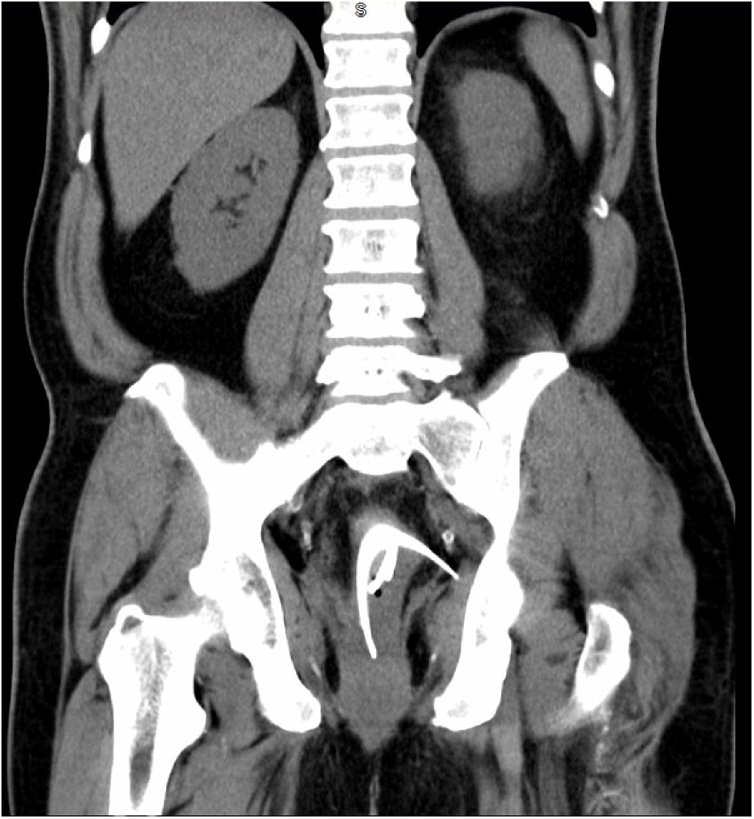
Coronal CT revealing multiple fragments of glass. One portion of the glass appeared to be traversing the inferior left lateral rectal wall, which was suspicious for perforation, however, no free air was present.
